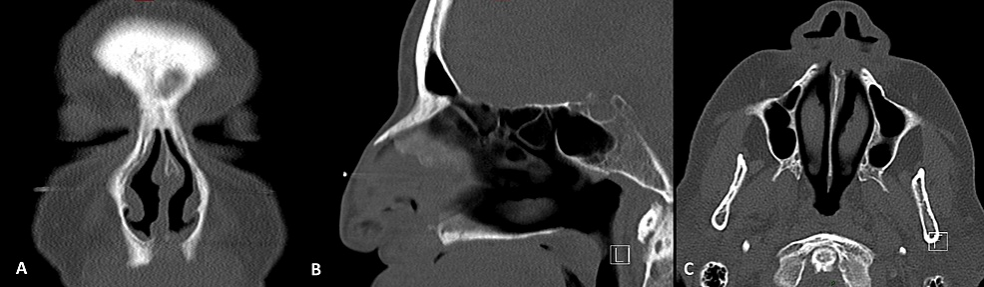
Postoperative paranasal sinuses CT scan. Absence of the calcified lesion at post-operative paranasal sinuses CT scan after six months of follow-up in coronal (A), sagittal (B), and axial (C) views.
